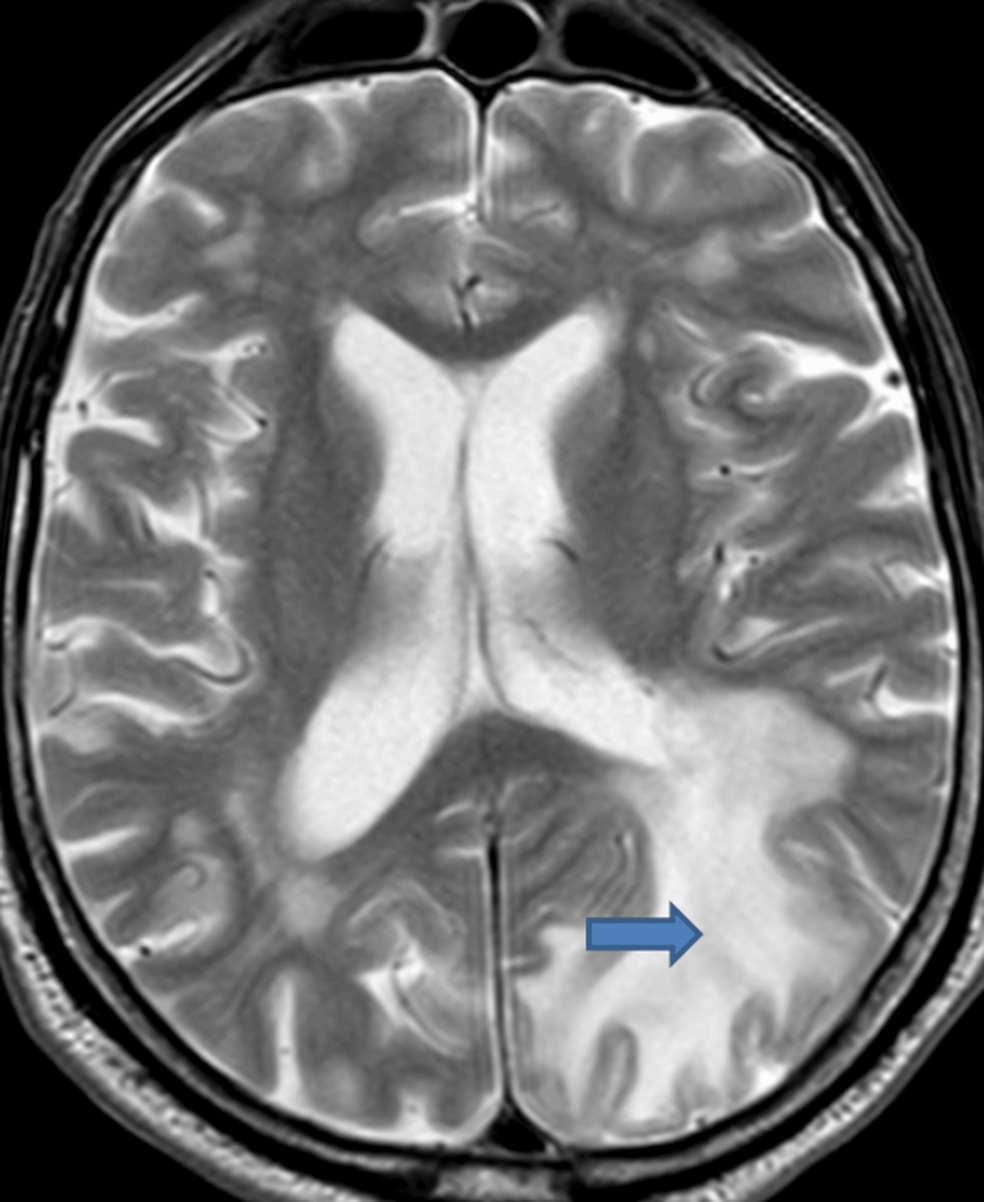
MRI head of the patient The arrow is pointing to an area of hyperintensity in the temporo-parieto-occipital lobe, likely suggestive of edema.
